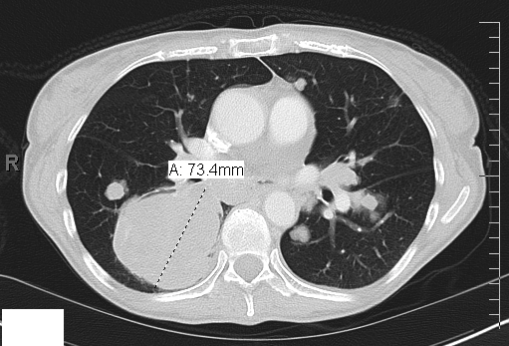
CT scan demonstrating progression of disease after second line palliative chemotherapy with carboplatin and docetaxel.
